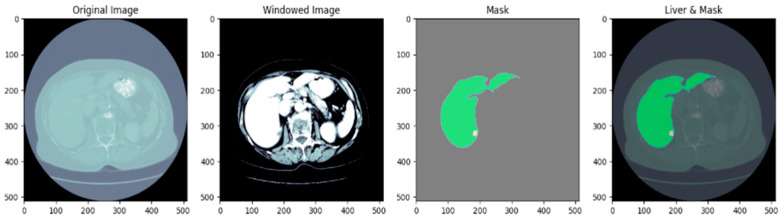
Preprocessing of CT scan: original image, windowed image, mask, liver, and mask overlay.
